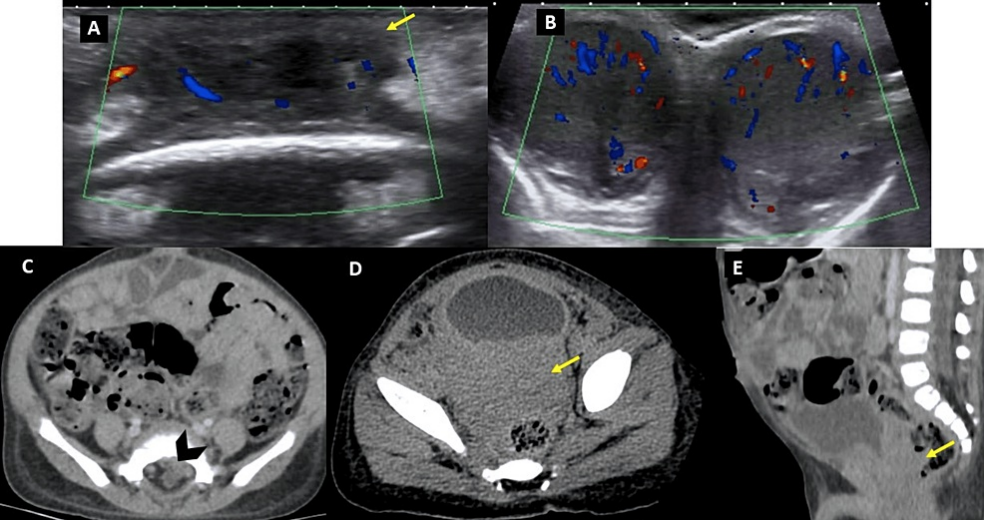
(A) Soft tissue ultrasound of the right frontal region showing a solid, hypoechoic mass with increased color Doppler flow, located in the subcutaneous tissue (yellow arrow). (B) Testicular ultrasound showing increased volume and color Doppler flow in both testicles, with hypoechogenicity of the parenchyma. (C) Axial abdomen and pelvis CT scan showing a solid mass in the left extradural intra-spinal space at the S1 level (yellow arrow). (D,E) Axial and sagittal abdomen and pelvis CT scan showing a solid mass without contrast enhancement located retrovesically with vesicoprostatic infiltration (yellow arrows).
